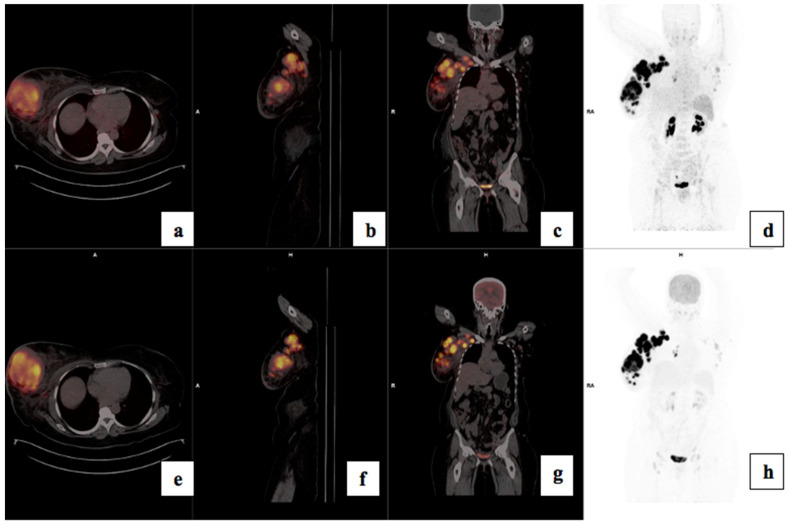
Shows ^68^Ga-Pentixafor (a–d) and FDG (e–h) images of a 56-year-old with TNBC T4bN2M0, Bloom Richardson Grade III Ki67 50%, showing intense uptake on both tracers in the right breast (a,e) and axillary lmph nodes (b,f). SUVmax was 36.85 on ^68^Ga-Pentixafor and 77.94 on ^18^F-FDG. The TBRs for FDG and Pentixafor were 19.5 and 17.4 in the primary and 7.6 and 7.2, respectively, in the axillary lymph nodes. The patient also has HIV with reactive inguinal lymph nodes, demonstrating mild uptake on both tracers (d,h).
